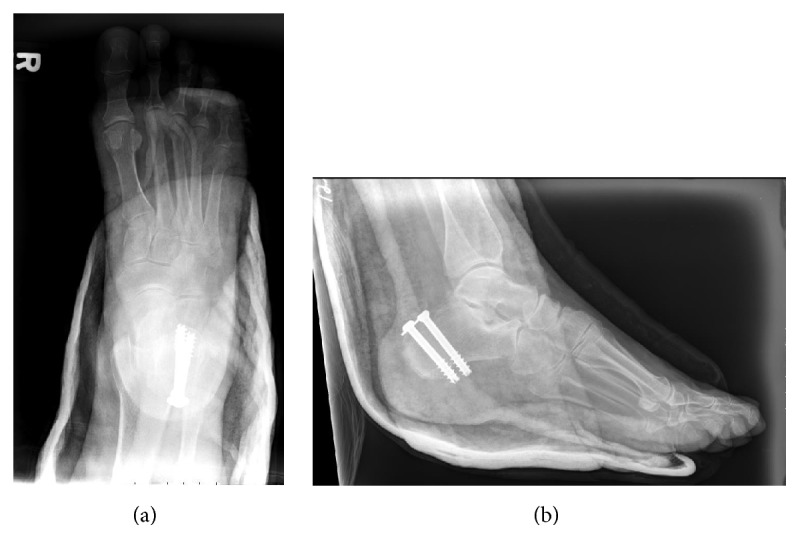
Anteroposterior (a) and lateral (b) radiographs taken immediately postoperatively.
